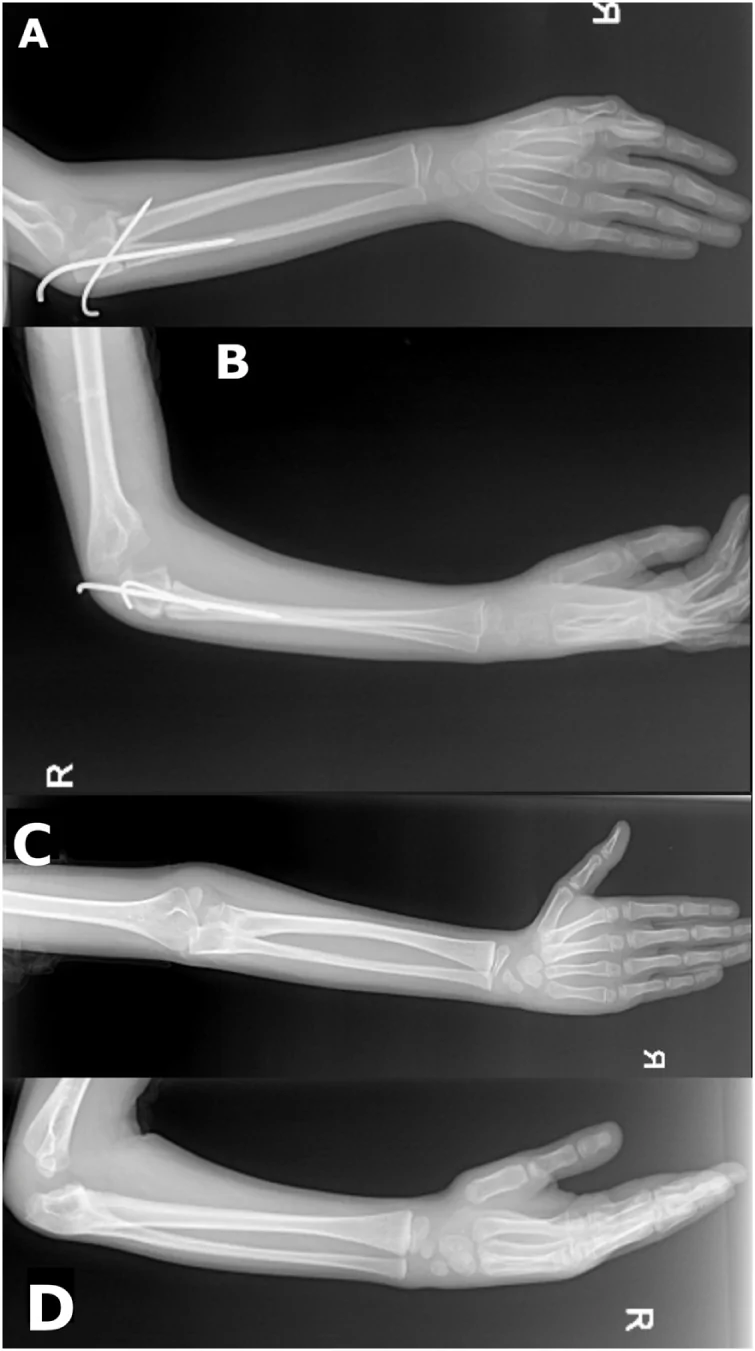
Postoperative radiographic findings. (A) Anteroposterior view at 4 weeks, demonstrating maintained correction with crossed Kirschner wires in situ and early callus formation at the osteotomy site. (B) Lateral view at 4 weeks, confirming satisfactory alignment. (C) Anteroposterior follow-up radiograph showing progressive union. (D) Lateral follow-up radiograph demonstrating complete radiographic union with satisfactory alignment.
